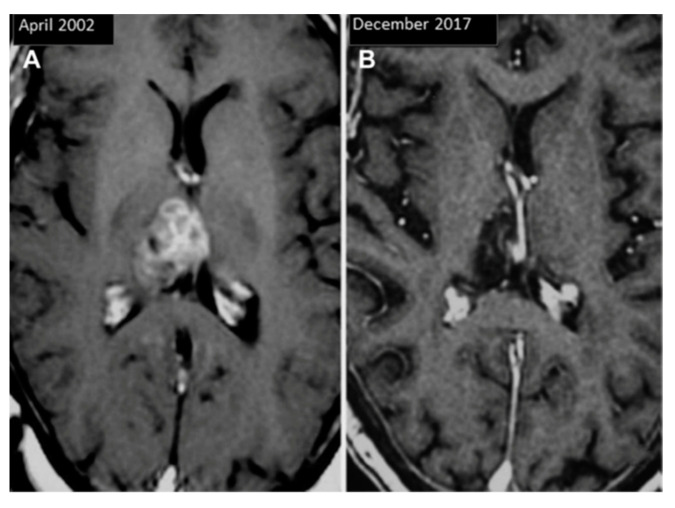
Pineal papillary tumor MRI before (A) and after 15 years of treatments (B). Reprinted with permission from Fernández-Mateos et al. (2018) [90]. Elsevier—2021 (License Number: 5034870401490).
